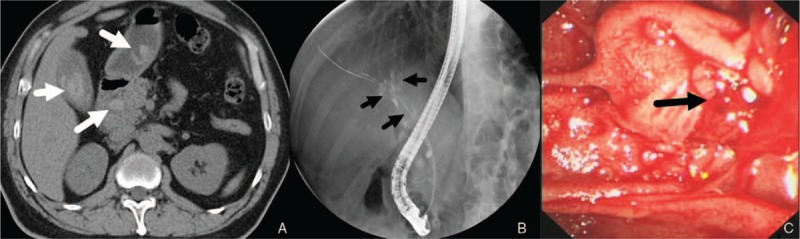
CT scan and ERCP. High-density masses with the suspicion of blood clots were showed in gallbladder, stomach and common bile duct ([A] marked with black arrows). Multiple filling defects in the common bile duct were showed on ERCP ([B] marked with white arrows). Bleeding from papilla of Vater could be directly seen during ERCP ([C], marked with black arrow). CT = computed tomography, ERCP = endoscopic retrograde cholangiopancreatography.
